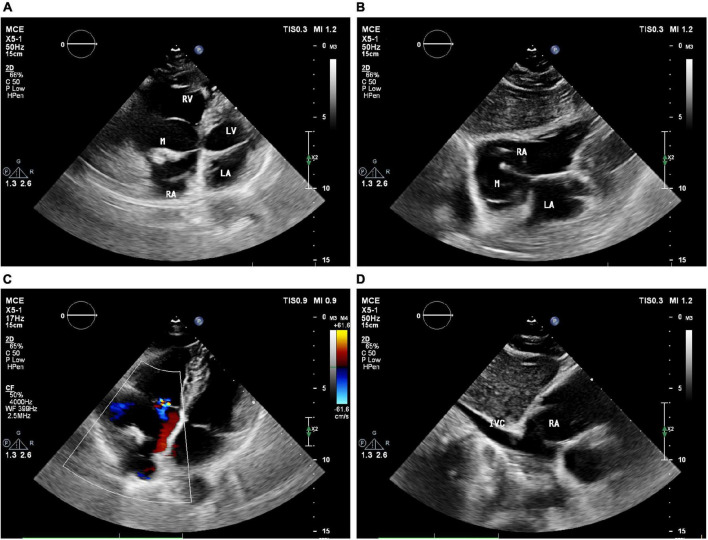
Echocardiography showed a large cystic-solid mixed echogenic lesion in the right atrium. Apical four-chamberview (A) and subxiphoid four-chamber view (B) showed that the mass was attached to the right atrial surface of the atrial septum. Ultrasound findings of tricuspid valve orifice (C) andinferior vena cava (D). M = (intracardiac mass).
